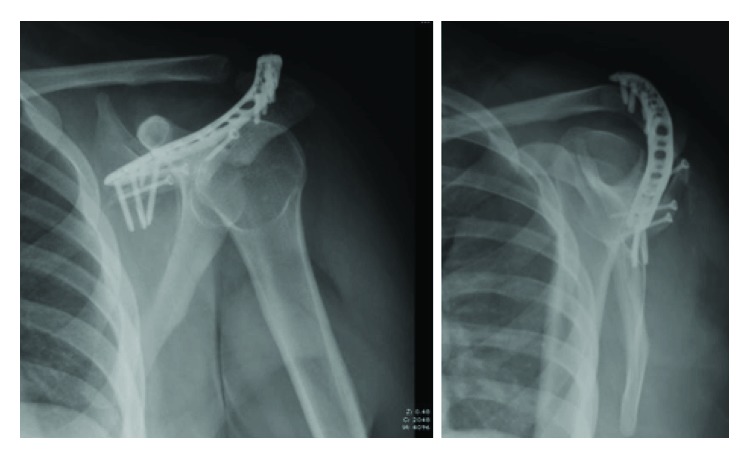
AP and lateral radiographic views of the scapula showing our fracture in appropriate alignment with evidence of implant failure or loosening.
